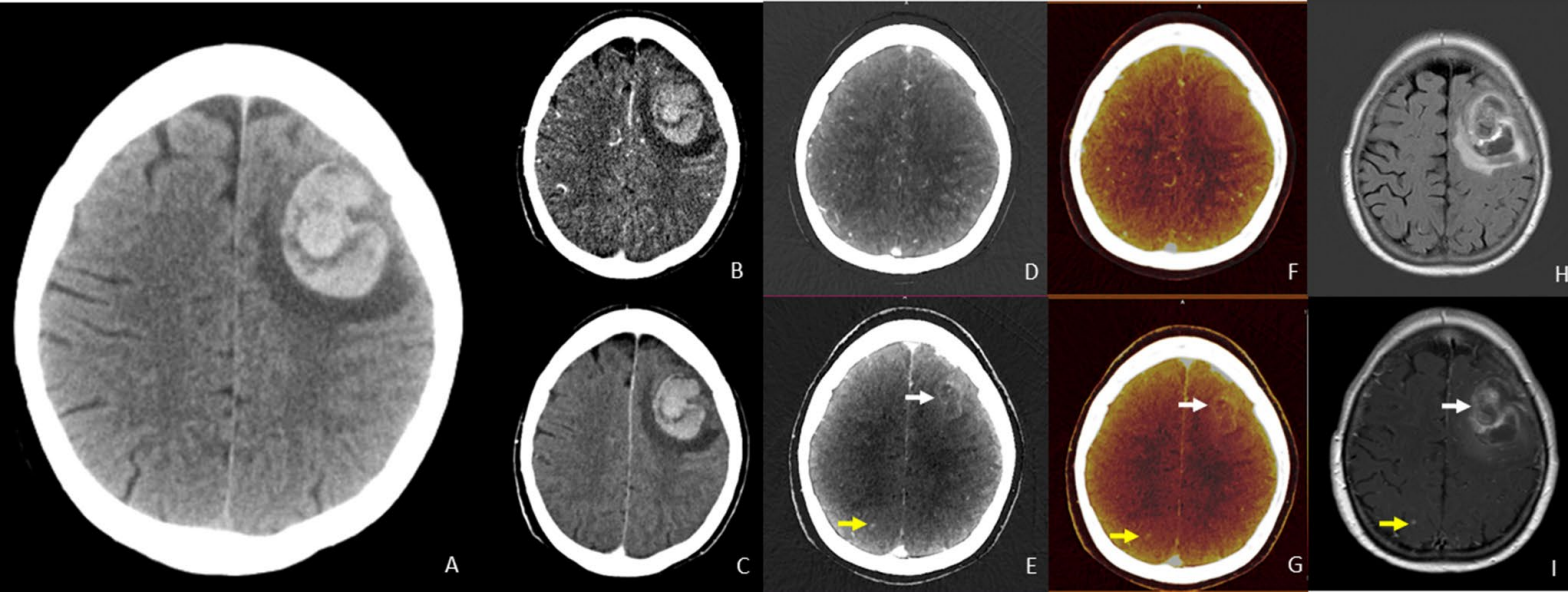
Left frontal ICH with underlying metastasis of a non-small-cell lung carcinoma in a 69-year old patient who presented with acute headache and aphasia. (A) The non-contrast CT shows left frontal intracerebral hemorrhage with surrounding edema. (B-C) Conventional-like CTA arterial and delayed phase images. (D-F) Arterial phase iodine – and fusion images. (E-G) Delayed phase iodine and fusion images show a ring-enhancing lesion (white arrow) suspected for metastasis, which was confirmed on fluid attenuated inversion recovery (FLAIR) and contrast enhanced MR images (H-I). The yellow arrow points to a contralateral enhancing lesion on delayed phase iodine and delayed phase fusion imaging as well as MR imaging, indicating a small contralateral cerebral metastasis.
